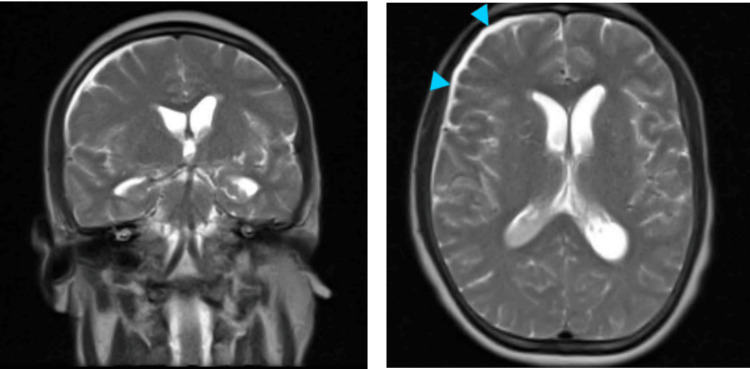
T2 coronal (A) and axial (B) reconstruction of brain MRI demonstrating a mild hydrocephalus of the body and inferior horns of the lateral ventricles. Right frontal subsegmental convexity fluid with CSF signal and mild mass effect is noted (blue arrowheads). CSF: cerebrospinal fluid
